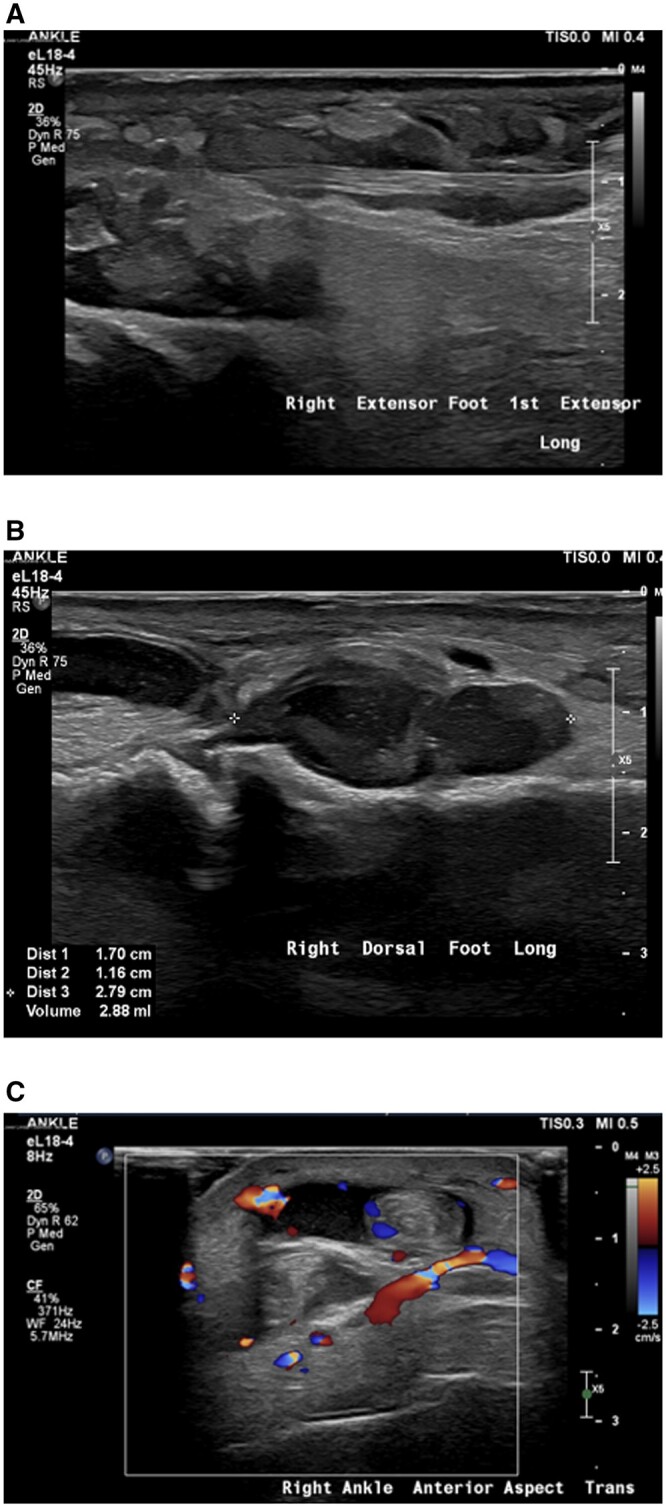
Ultrasound images demonstrate the appearance of subcutaneous panniculitis. (A, B) Ultrasound of the right dorsal foot demonstrates innumerable subcutaneous nodules of echogenic fat pad in keeping with panniculitis. (C) Ultrasound of the right ankle demonstrates fluid in the tendon sheaths and increased vascularity consistent with tenosynovitis of the extensor hallucis longus and digitorum longus tendons.
